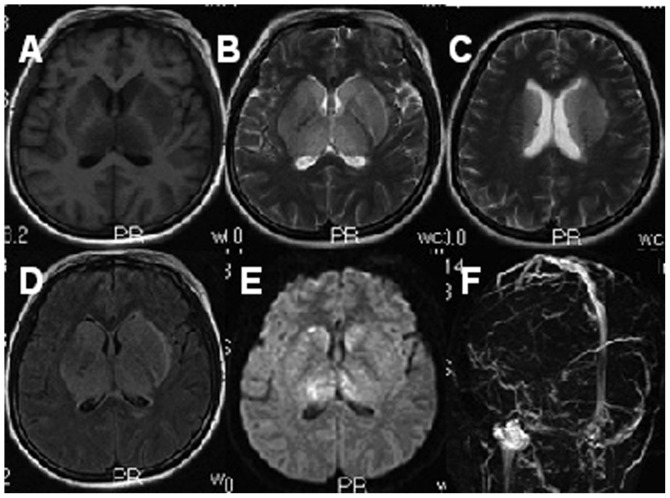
(A–C) Long T1 and T2 signals with hyperdense FLAIR signals in the bilateral thalamus and basal ganglia regions according to MRI, as indicated by the arrows; (E and F) non-seriously restricted diffusion according to diffusion-weighted imaging; (F) inferior sagittal and straight sinus thrombosis.
